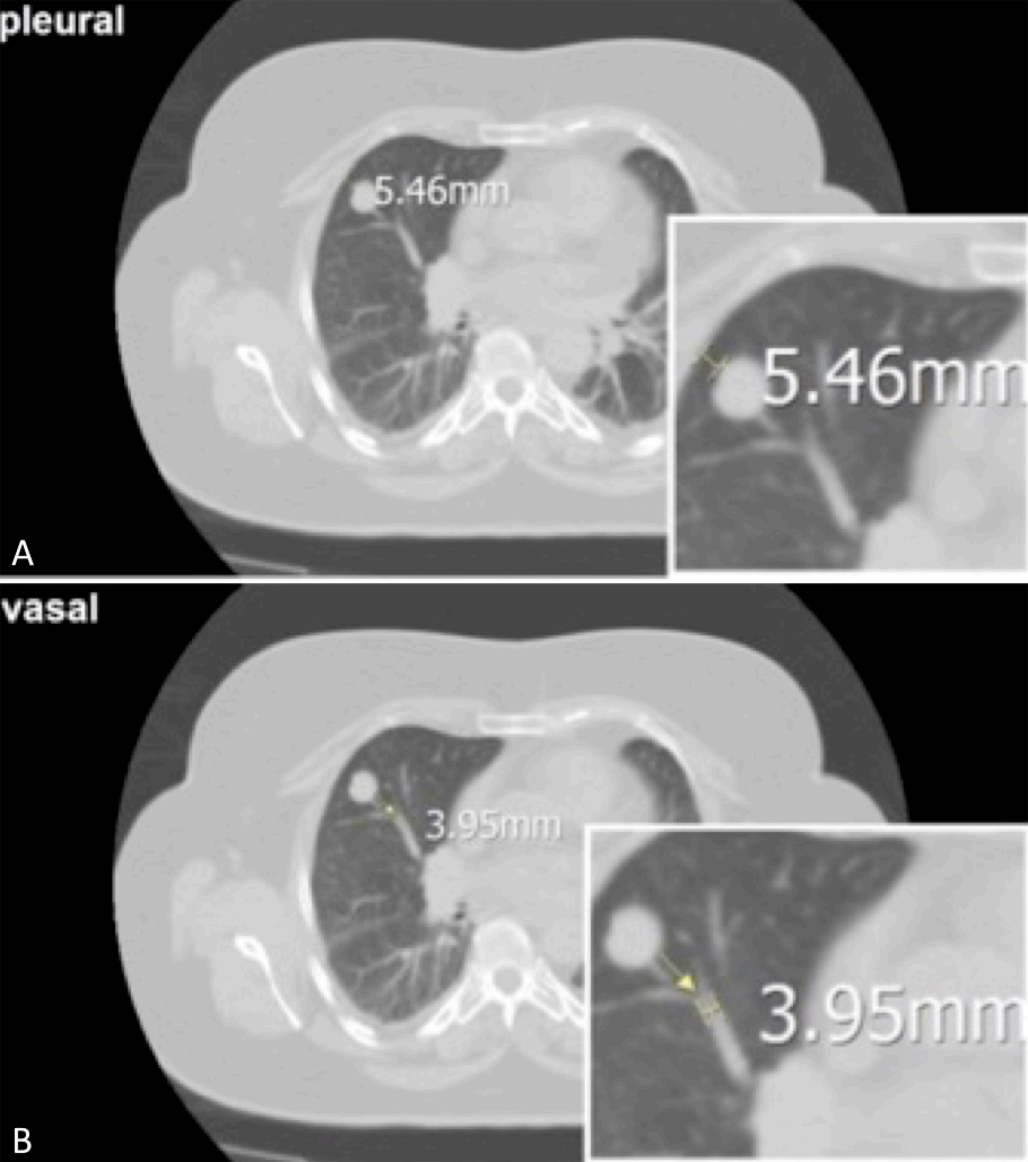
(A) The axial CT scan of a pulmonary lesion shows the distance to the pleura (5 mm) as a possible influence. (B) and the distance to an adjacent vessel (4 mm).
